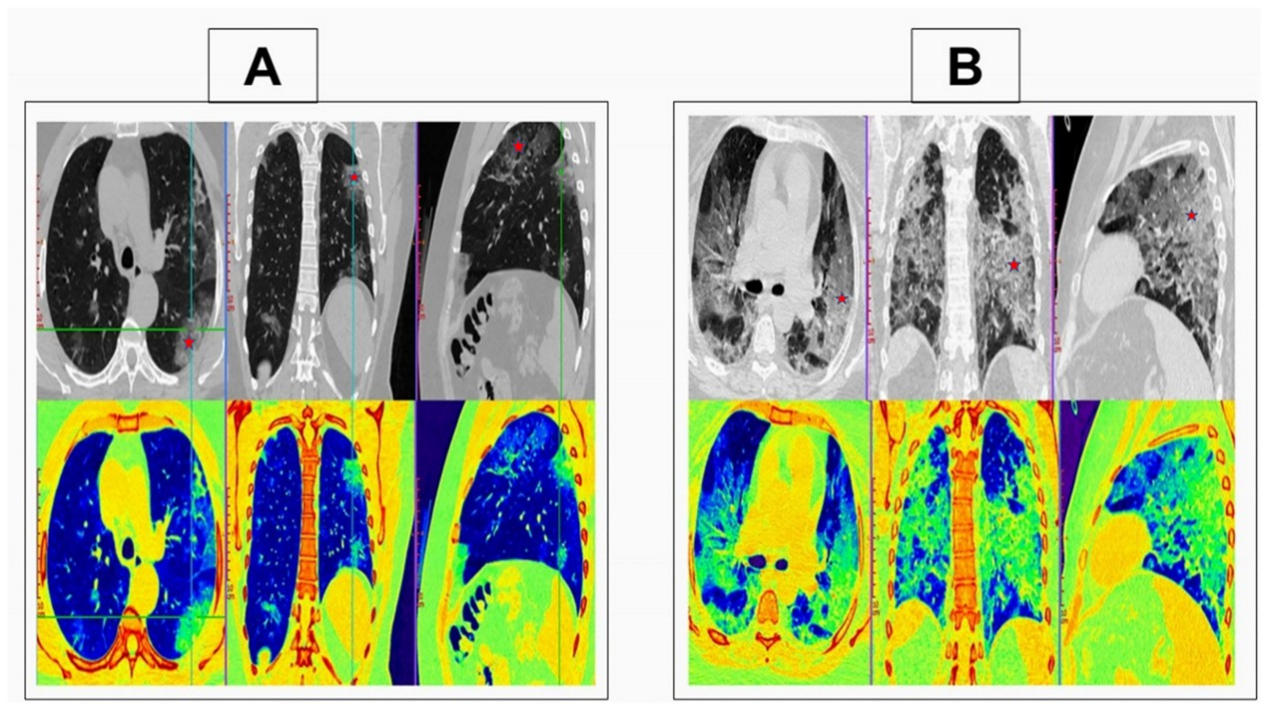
CT scan chest with multi-planar and color-coded images. (A) A 44 years old male patient presented with fever and cough. CT scan chest with multi-planar and color-coded images showed bilateral multi-focal patchy ground-glass opacities with a peripheral subpleural predominance (asterisk). CT severity score was 7. The patient exhibited a mild disease course. (B) A 30 years old male patient presented with fever, dyspnea, and cough. CT scan chest with multi-planar and color-coded images showed widespread bilateral central and peripheral confluent ground-glass opacities (asterisk). CT severity score was 21. The patient exhibited a severe disease course.
